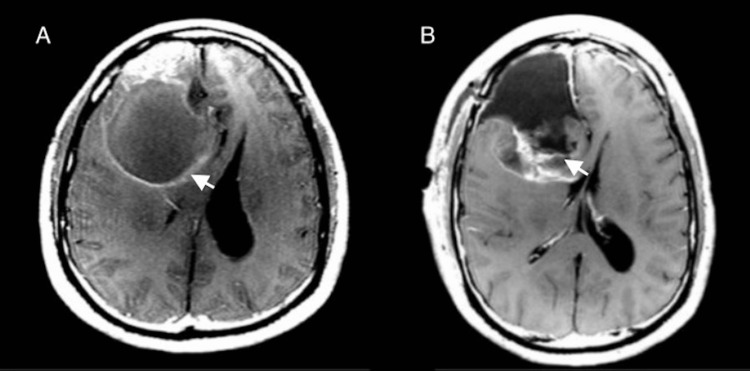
Right frontal oligodendroglioma A: Initial preoperative axial postcontrast T1-weighted MRI revealed a large right frontal lobe mass associated with focal calcifications and possible blood products suggestive of oligodendroglioma with a midline shift to the left. B: Postoperative MRI after initial surgery presents gross total resection of the tumor with a persistent mass effect 9 mm leftward midline shift.
